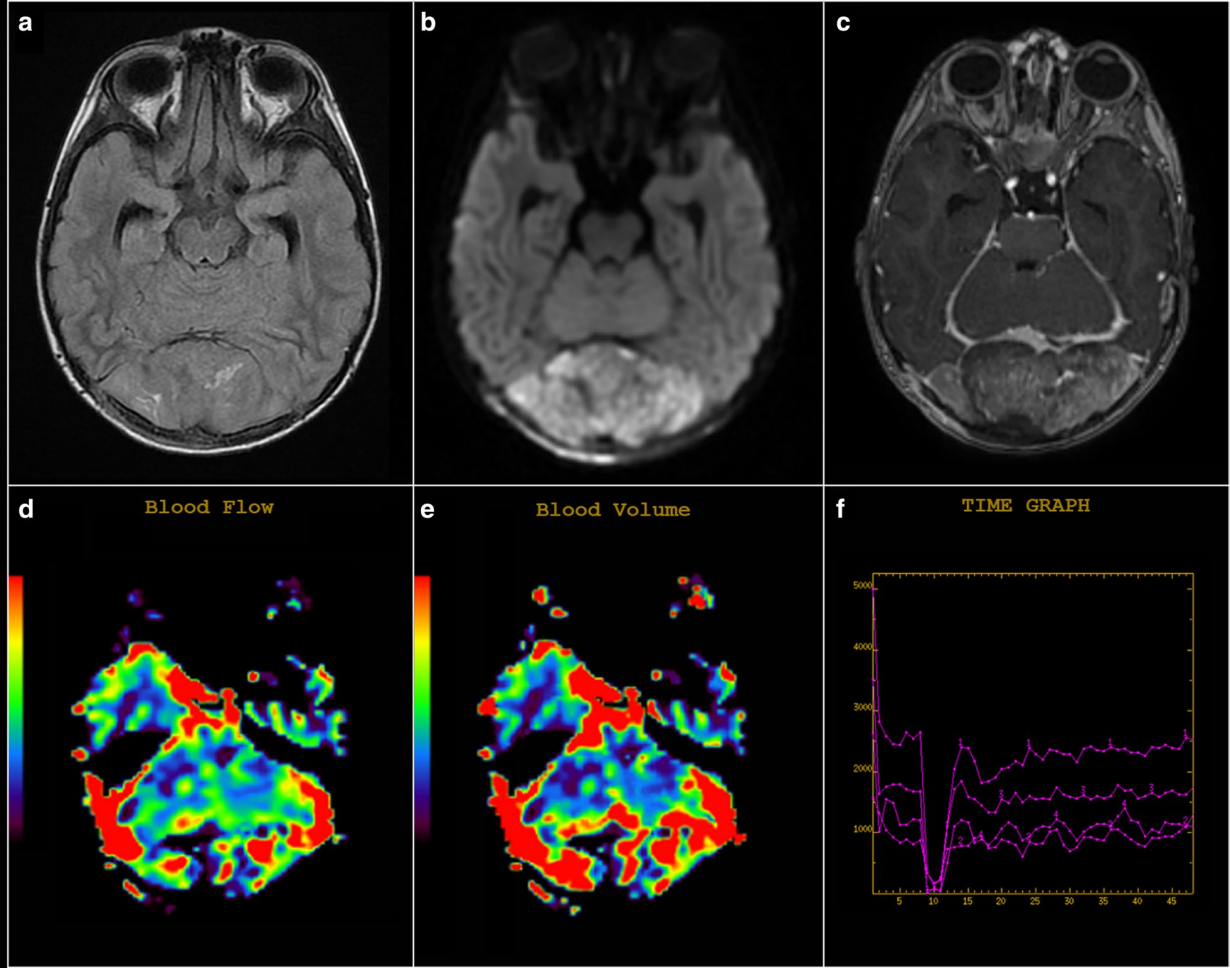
Advanced MRI imaging for medulloblastoma. Axial FLAIR (a) and DWI (b) display an extra-axial mass occupying the lateral and retrocerebellar regions, demonstrating a high signal in FLAIR with restricted diffusion in DWI. Axial T1 after contrast administration (c) demonstrates strong heterogeneous contrast enhancement of the mass. DSC-MR perfusion maps (d–f) show increased vascularization in the mass, with increased blood flow (d) and high value of rCBV (e) of the mass than the normal parenchyma. *DWI* diffusion-weighted image, *DSC* dynamic susceptibility contrast-enhanced, *rCBV* relative cerebral blood volume
